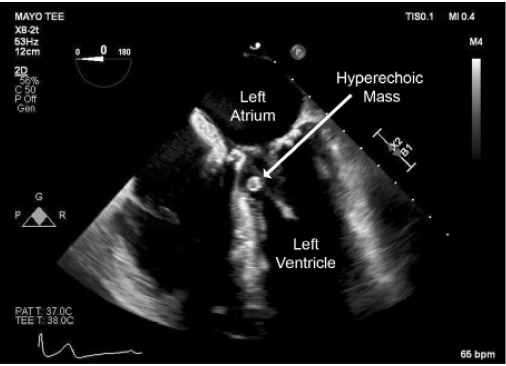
Transesophageal echocardiogram midesophageal long-axis view demonstrating a hyperechoic mass near the mitral annulus (arrow).
